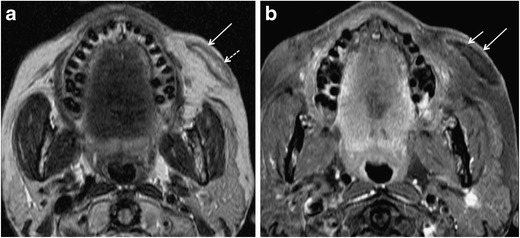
61-year-old woman with discrete facial asymmetry and induration of the left cheek. She had internal fixation of a left tetrapod facial fracture 25 years back. MRI showed a well-circumscribed lesion, isointense to fat on T2 W (a) and post-gadolinium T1 W fat saturated (b) sequences (*long arrows*). The lesion is located in the left superficial medial cheek fat. Patient confirmed autologous fat filler injection nine months back. The hypointense rim around the filler deposit on T2 and the post-gadolinium rim enhancement on T1 represent a fibrous pseudo-capsule due to scar tissue formation: *dashed short arrow* in (a) and *short arrow* in (b)
